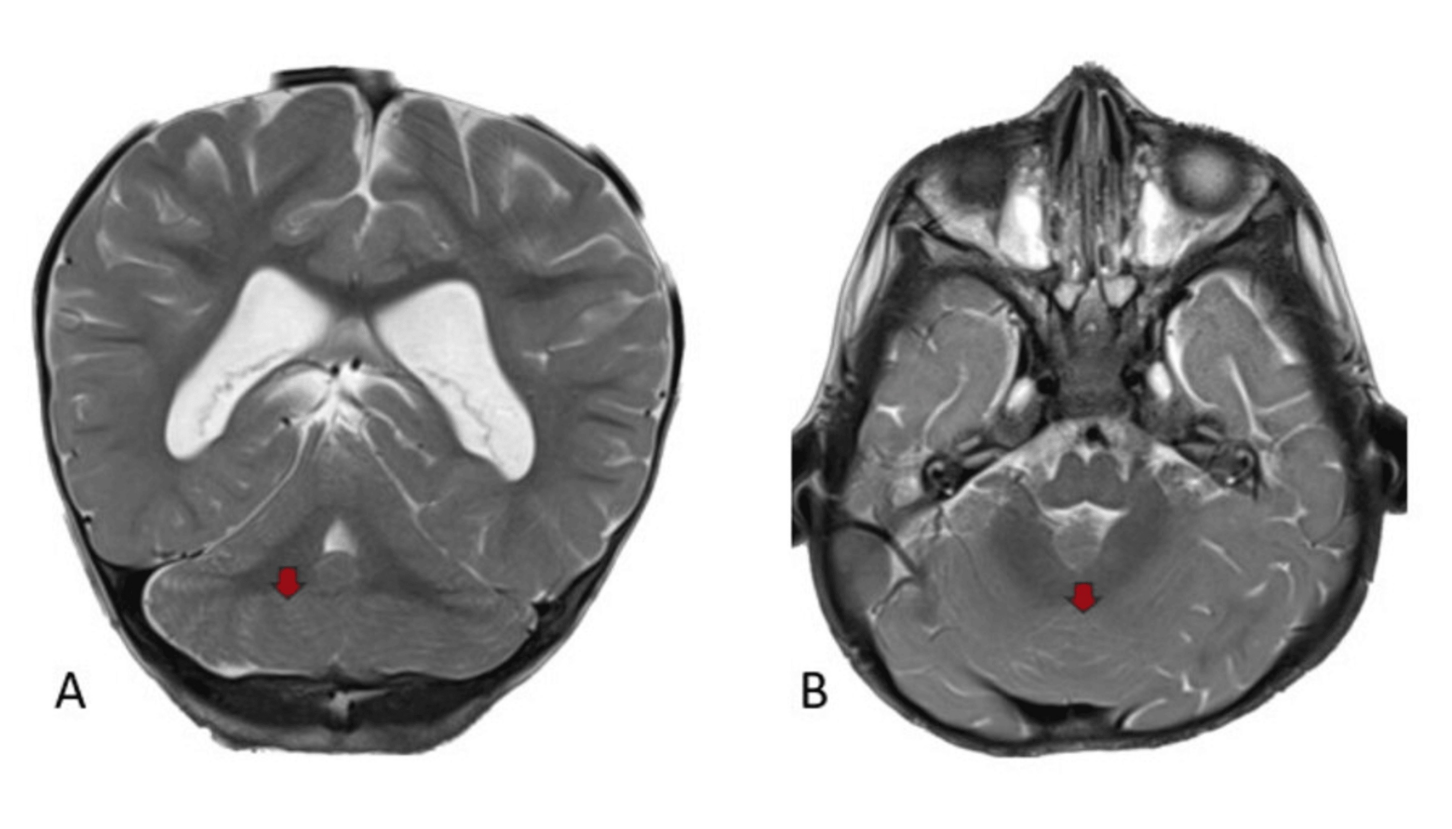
MRI T2WI findings A (coronal) and B (axial) views reveal uninterrupted continuity of white matter and folia across the midline (red arrows), indicative of a single-lobed cerebellum with transversely oriented folia, confirming fusion MRI: magnetic resonance imaging; T2WI: T2-weighted imaging
